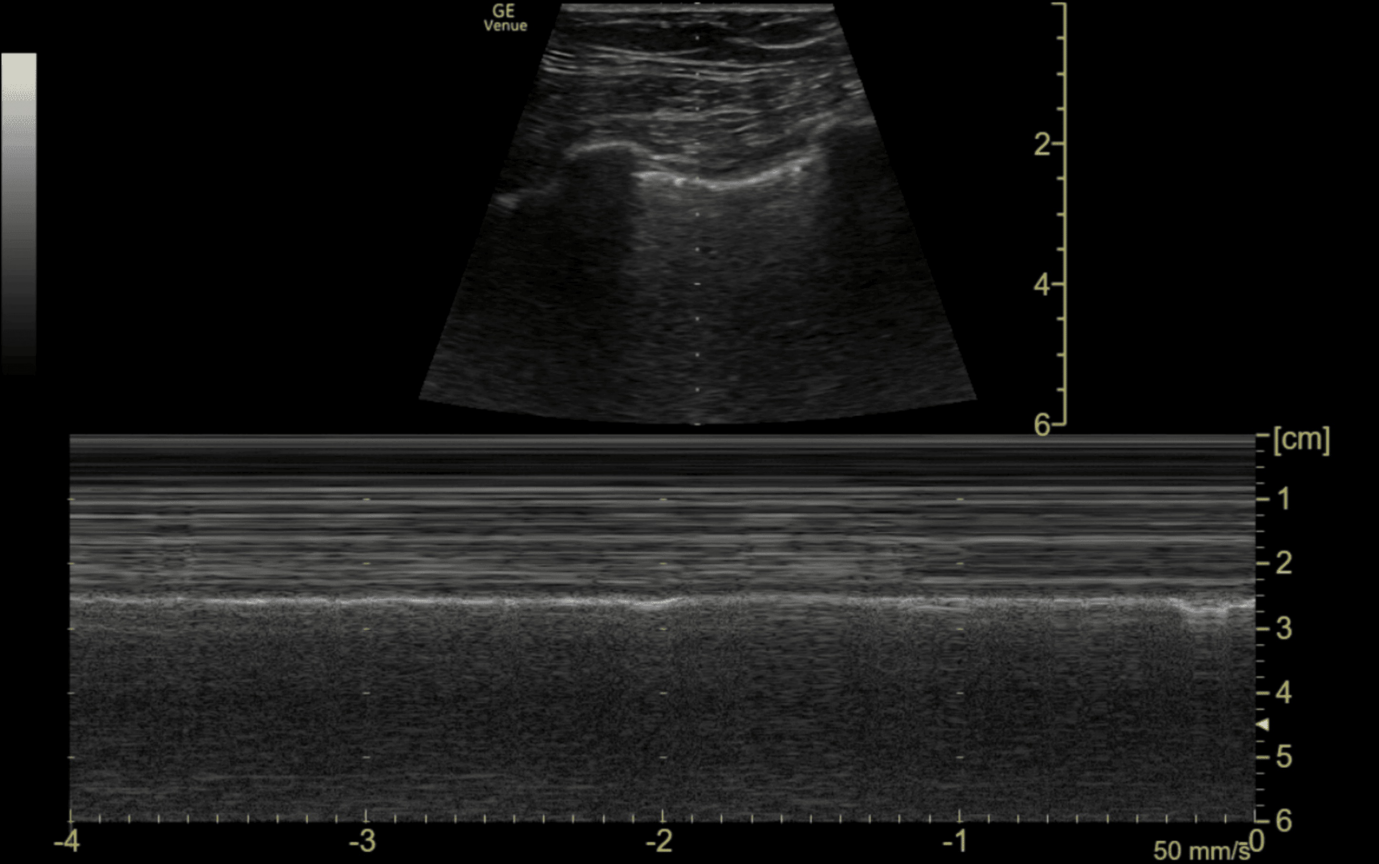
Lung ultrasound of the left lung. B-mode and M-mode images demonstrate normal lung sliding with a seashore sign, excluding pneumothorax
